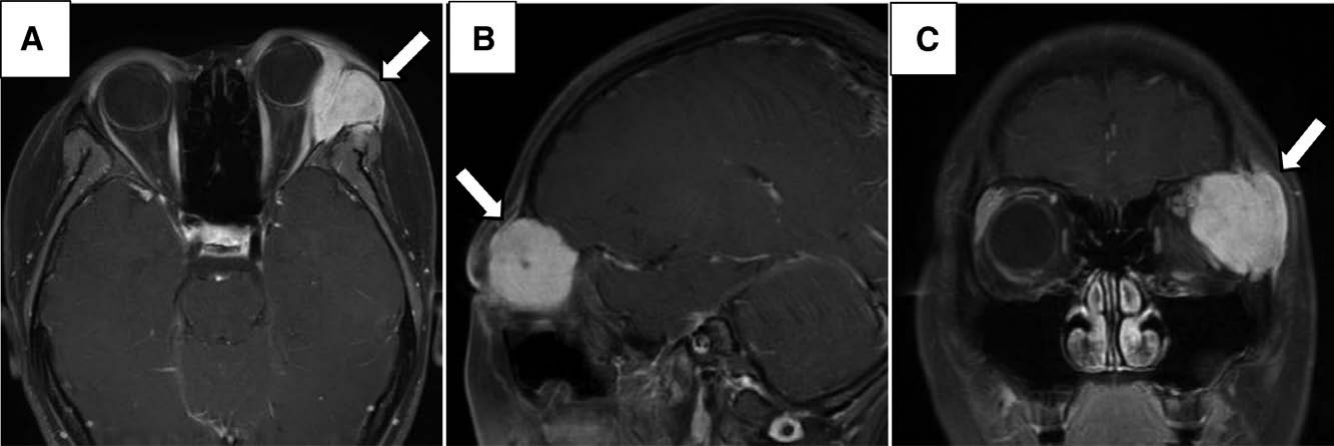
MRI from axial (A), sagittal (B), and coronal (C) views revealed an irregular mass in the left lacrimal gland region, about 3*3.2*3.2 cm in size. Left anterior process, adjacent external rectus muscle compression displacement, left orbital wall bone destruction. MRI = magnetic resonance imaging.
